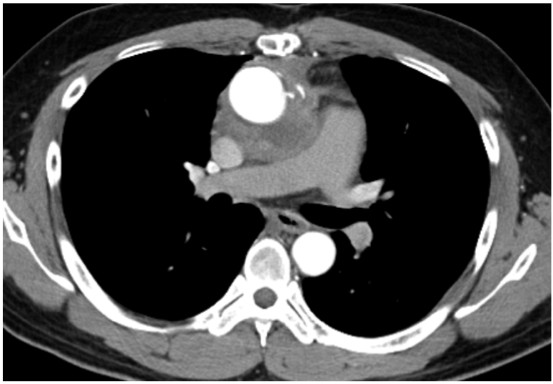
Postoperative chest CT. No compressions were observed at the left main bronchus and the right pulmonary artery after total aortic arch replacement.
